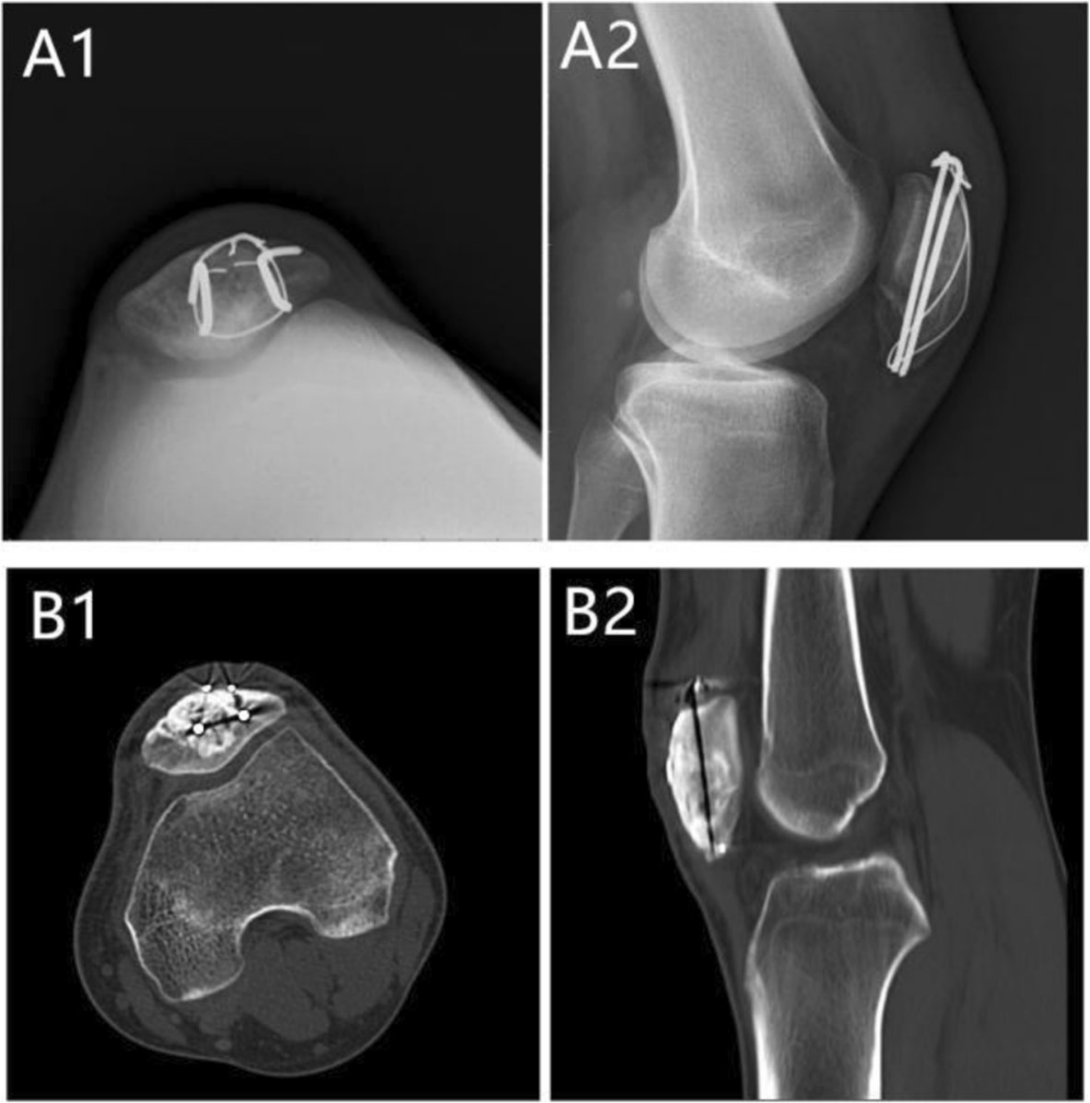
X-ray and CT scan obtained at 21 months after the operation. A, B A1 and A2 Axial images of the patella and lateral X-ray films of the knee joint showed that the grafting bone had been completely incorporated with the host bone, and the fixation showed no looseness or rupture. B1 and B2 Cross and sagittal CT scans revealed bony union
